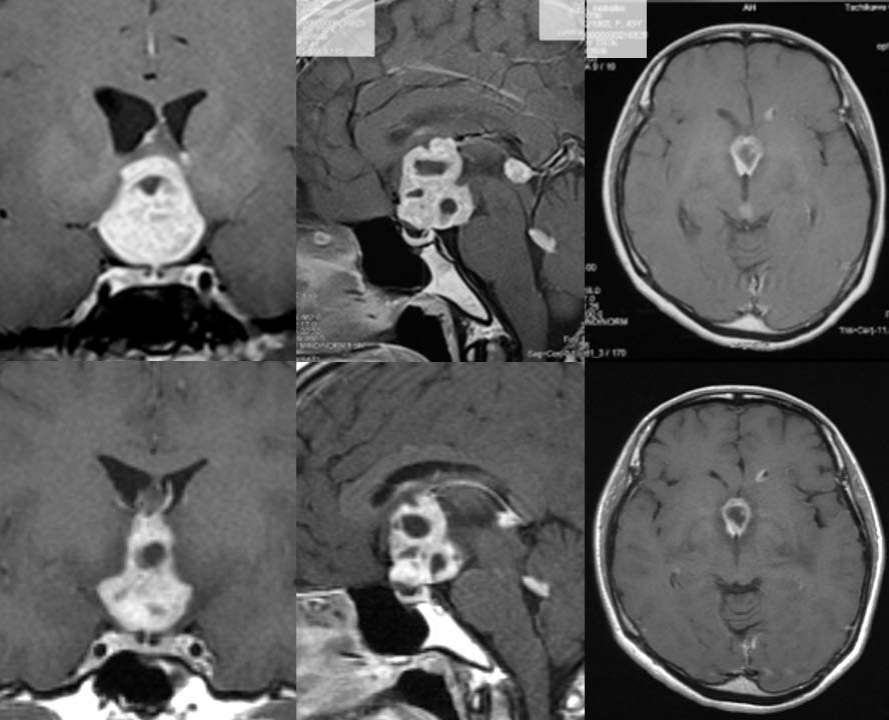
Contrast-enhanced MRI showing multiple enhanced lesions in the third ventricle, pineal region, and fourth ventricle (upper).
